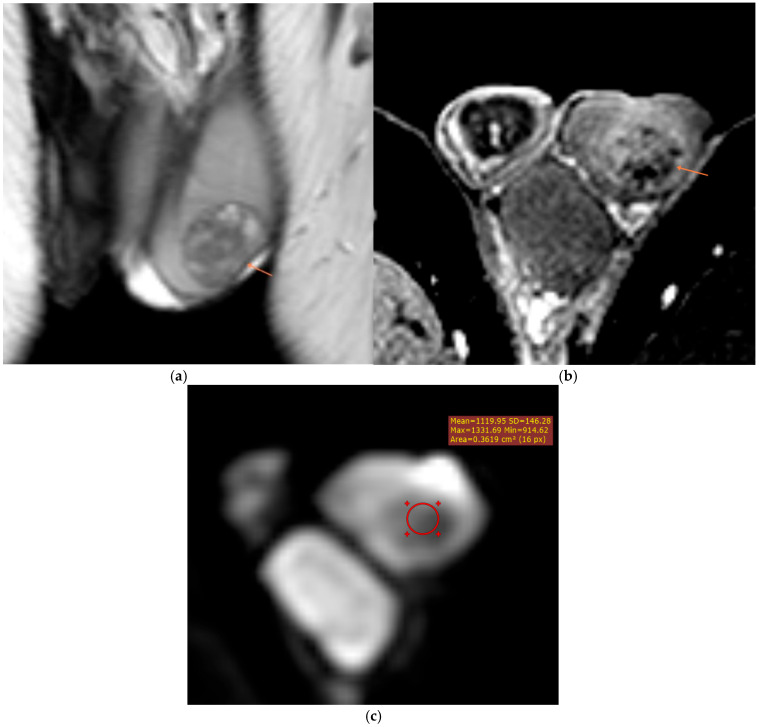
Mixed germ cell tumor of the left testis. (a) Coronal T2-weighted image demonstrates an irregular intratesticular mass (orange arrow). (b) Axial post-contrast T1-weighted image shows heterogeneous enhancement with avascular hypointense regions representing necrosis. (c) ADC map demonstrates mild diffusion restriction within the tumor, with an ADC value of approximately 1.1 × 10^−3^ mm^2^/s.
